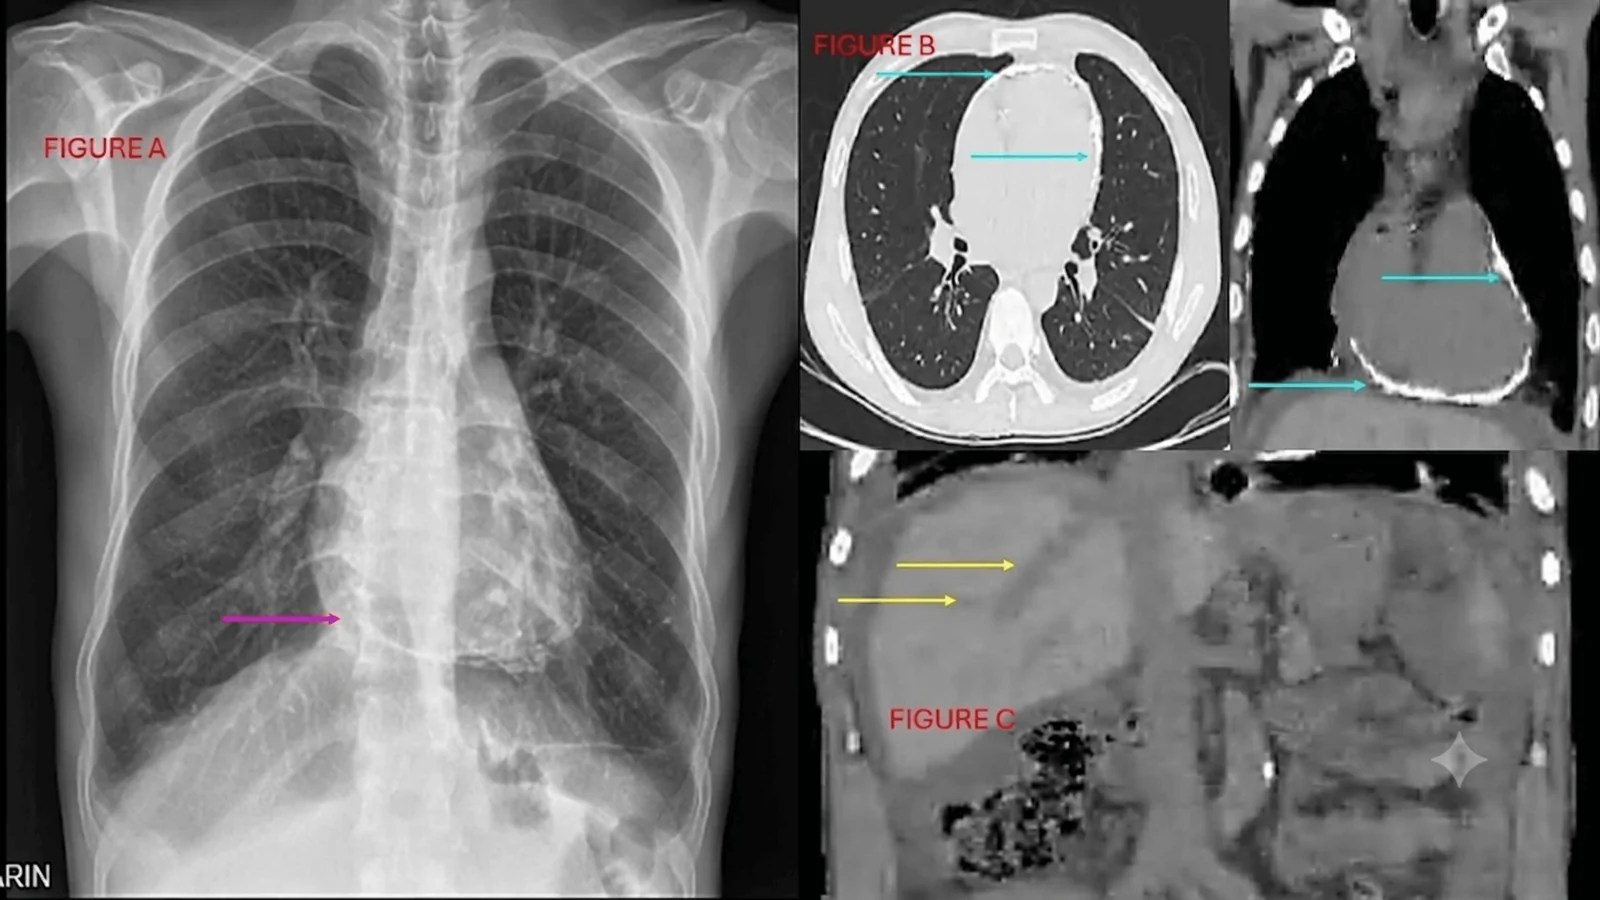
Multimodal diagnostic evaluation using thoracoabdominal imaging (A) Chest X-ray (posteroanterior (PA) view): The magenta arrow indicates a thin peripheral radiopaque line of pericardial calcification. (B) Chest CT (axial view): The cyan arrows show massive, circumferential pericardial calcification ("armor heart"). (C) Abdominal CT: The yellow arrows point to the marked dilation of the hepatic veins due to high pressure.
